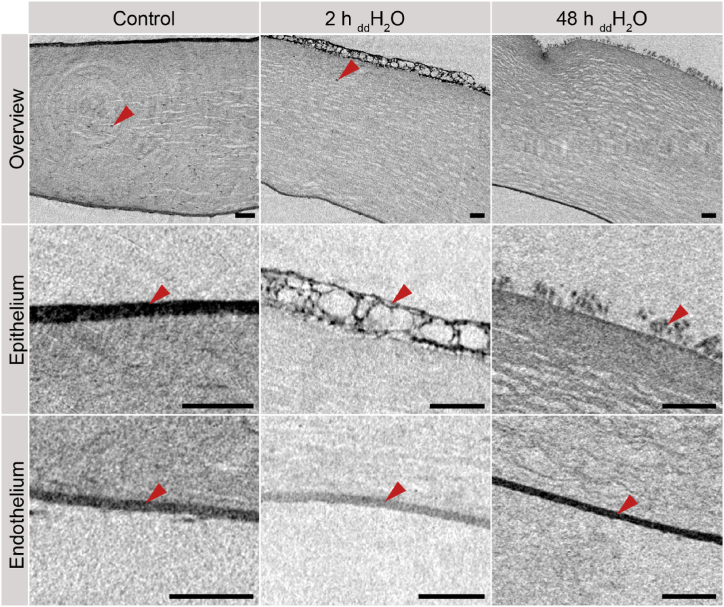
Visualization of the stroma and stromal cells (top row, arrow) corneal epithelium (middle row, arrow), and corneal endothelium (bottom row, arrow) using Nano-CT with iodine-based contrast enhancement. Scale bar: 100 μm.
